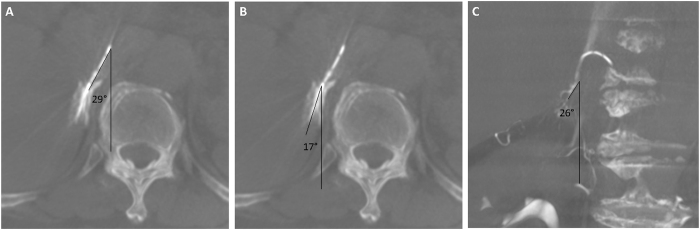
(a) A 56-year-old female with primary aldosteronism. Maximal intensity projection (3-mm thickness) of the Dyna CT images showed a well-opacified right adrenal gland. The catheter axial angle was 29°. (b) The venous axial angle between the right adrenal vein and the anteroposterior axis of the same patient was 17°. (c) Another 59-year-old male with primary aldosteronism. The venous coronal angle between the right adrenal vein and the vertical axis of the patient was 26° in this case.
